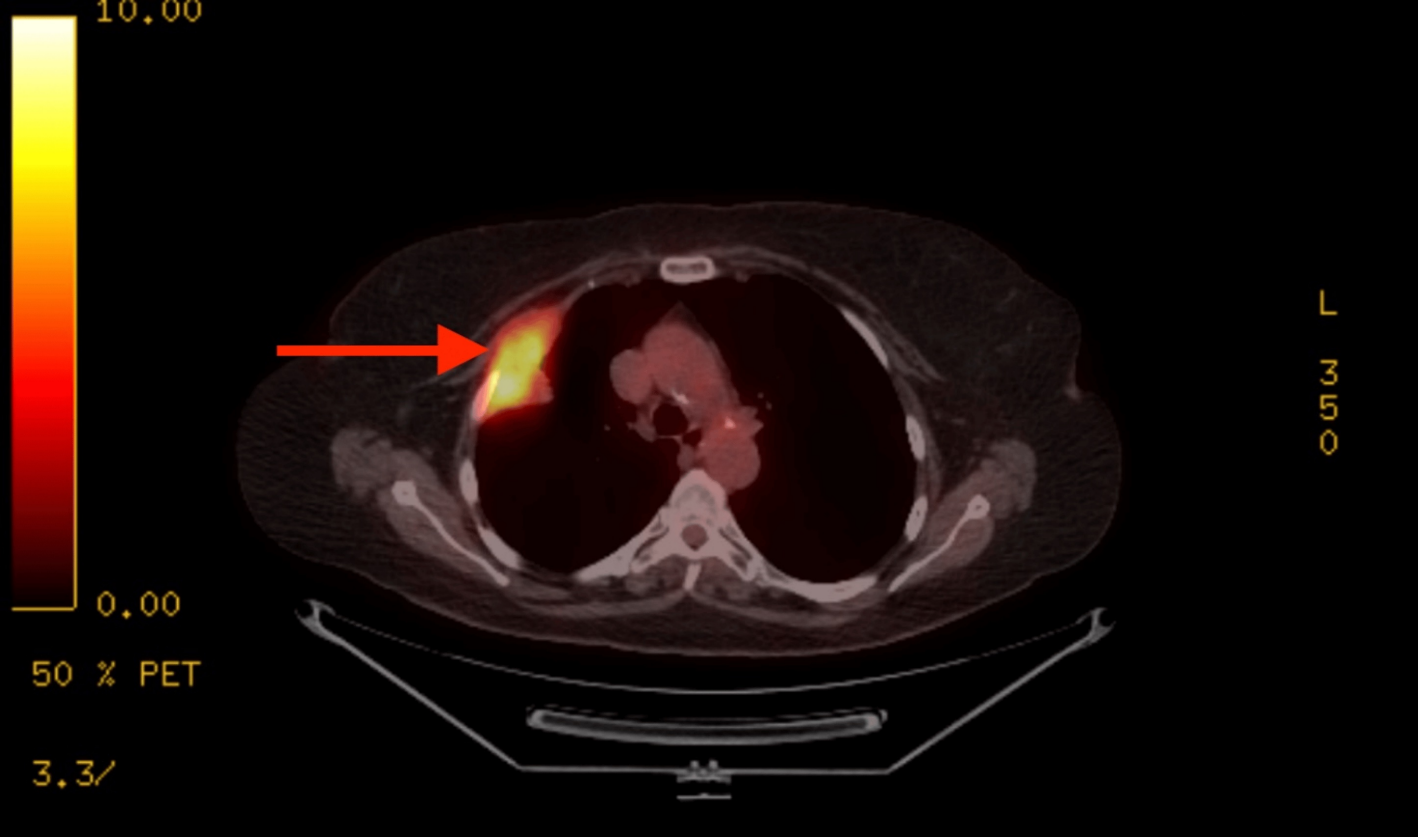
Positron emission tomography (PET) CT shows a large mass (arrow) within the right upper lobe extending to the chest wall and involving the second and third right ribs (Standardized Uptake Value 8.8 g/ml)
